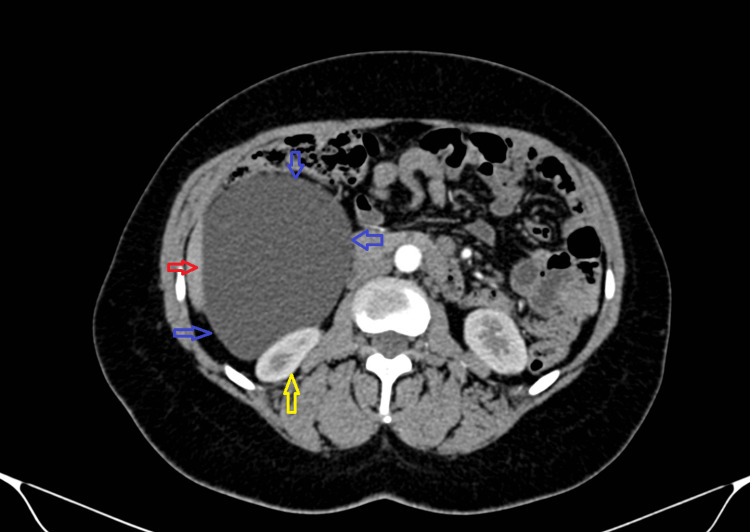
Axial CT image of abdomen and pelvis with intravenous contrast showing a (14x10x6cm) right retroperitoneal simple cyst (red arrow: liver; blue arrows: retro-peritoneal cyst; yellow arrow: right kidney) CT, computed tomography
